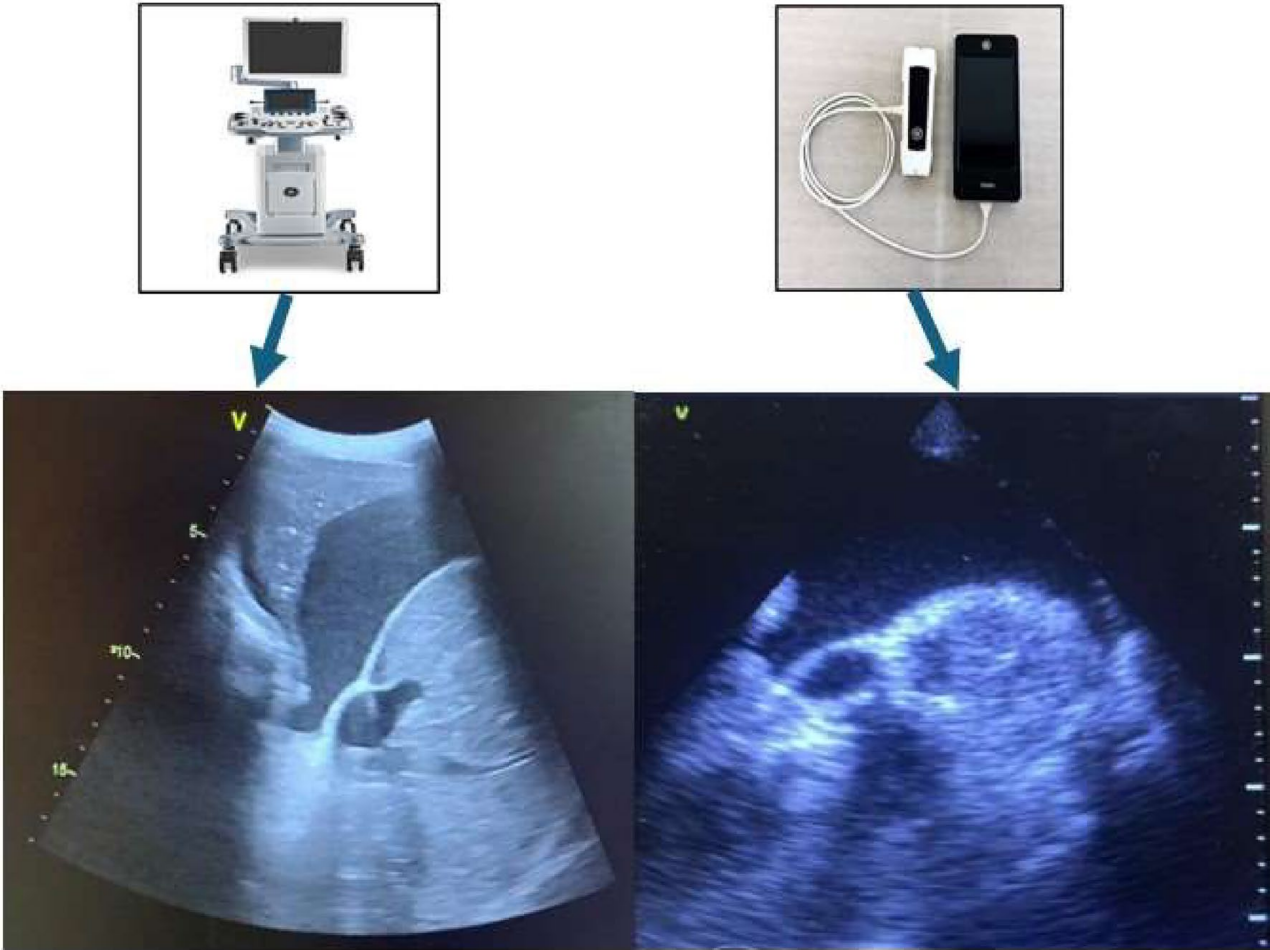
On the left, a pleural effusion detected using the high-end ultrasound machine; on the right, a pleural effusion highlighted with the handheld ultrasound device
